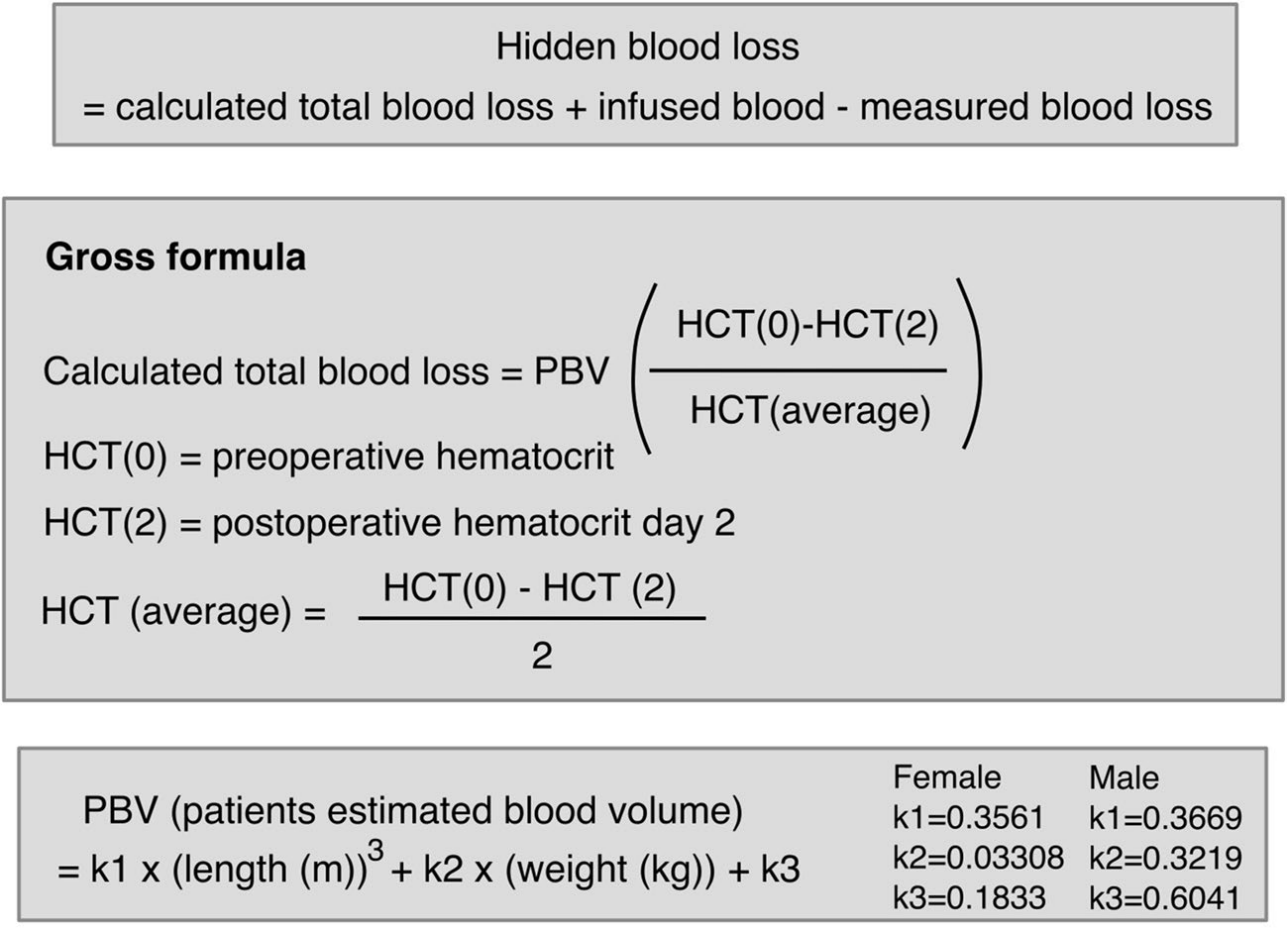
A 14-year-old boy with congenital kyphosis due to T10 posterior hemivertebra. Vertebral column resection of T10 via costotransversectomy approach, reconstruction with TLIF cage and instrumentation from T7 to L2 with intraoperative blood loss of 400 ml. Standing posteroanterior and lateral preoperative and 2-year follow-up radiographs
